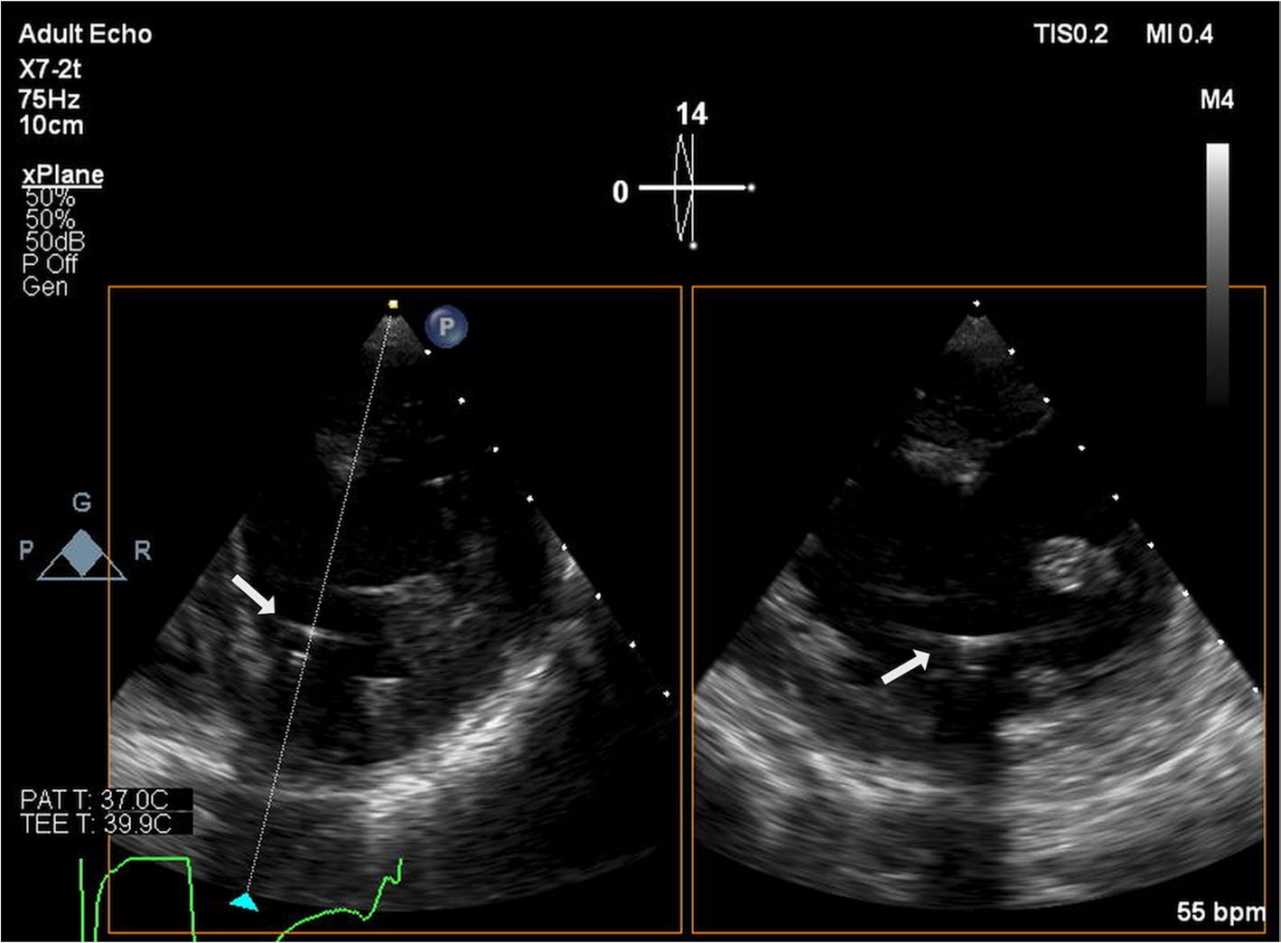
Transesophageal echocardiography images of pulmonary artery catheter malposition in the left ventricle. Biplane images were obtained using the transgastric approach. The left and right images are a short-axis view and a two-chamber view of the left ventricle, respectively. The pulmonary artery catheter balloon (white arrows) was inflated with air, allowing observation of the high-intensity reflection and the acoustic shadow behind it
